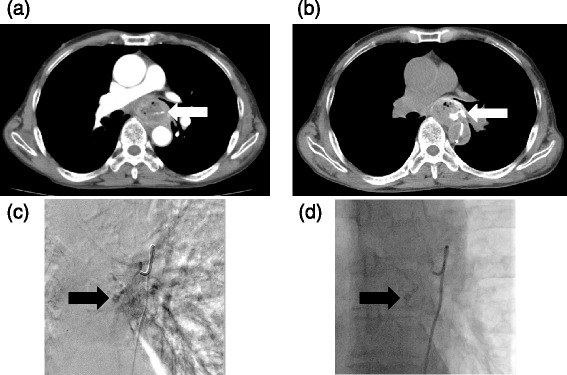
Arterial esophageal bleeding in case 2. a Enhanced CT shows that contrast material was in the esophagus in the arterial phase, indicating extravasation of contrast material (*arrow*). b Enhanced CT with angiography of the esophageal artery shows contrast material extravasation in the esophagus (*arrow*). c An image of digital subtraction angiography of the esophageal artery demonstrates punctate contrast material collections in the esophagus (*arrow*). d After transcatheter arterial embolization with a mixture of *N*-butyl cyanoacrylate (NBCA) and Lipiodol (*arrow*), active bleeding improved
